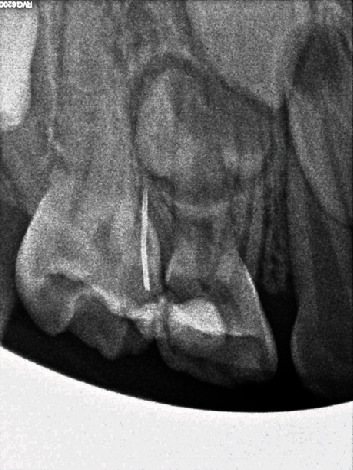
The periapical radiograph shows the exact location of a broken tool between teeth 55 and 54.
